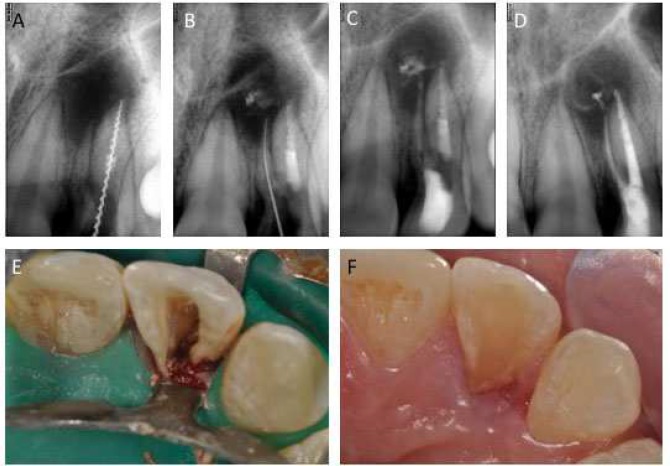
A)* Working length radiograph for the main canal; *B)* Working length radiograph for the accessory root; *C)* Intra-canal medicament; *D)* Post-obturation radiograph; *E)* Access cavity after obturation; *F)* Restored access cavity
